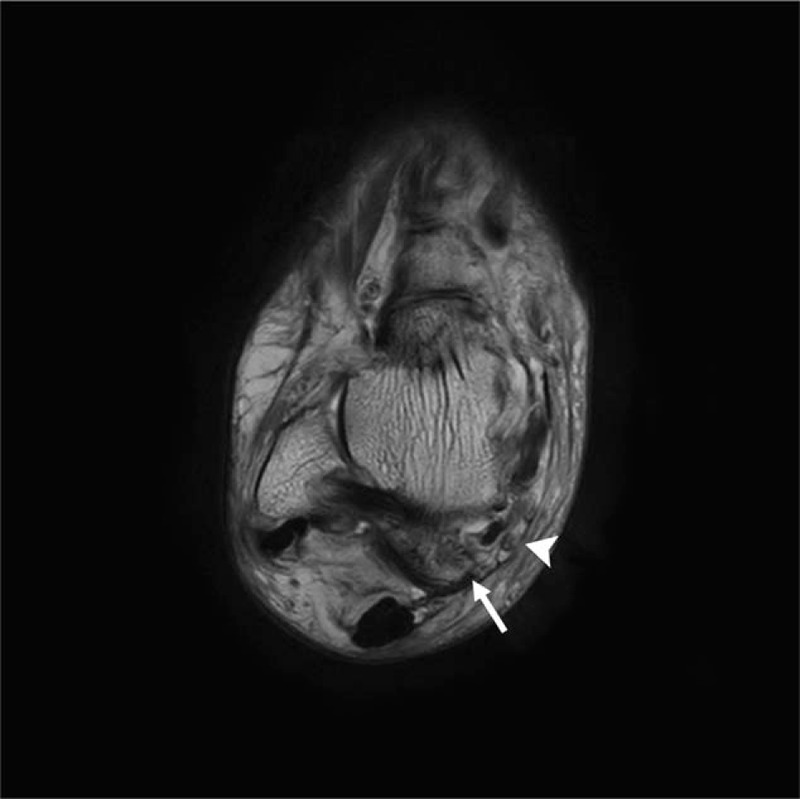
Preoperative axial T1-weighted magnetic resonance image of the right ankle showing an abnormal posterior talocalcaneal coalition (arrow) compressing the posterior tibial nerve (arrowhead).
